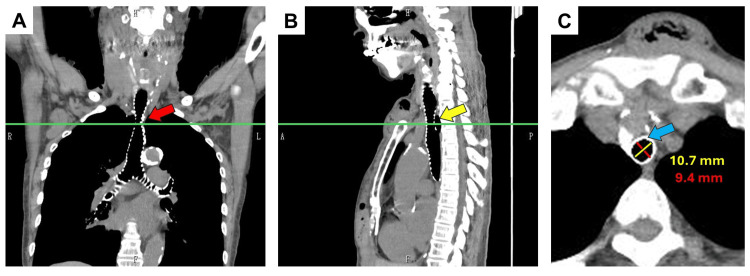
Postoperative computed tomography multi-planar reconstruction image of the present case. A: Coronal image of the trachea. Tracheal stenosis (red arrow) is observed. The green line indicates the level of the narrowest part of the trachea. B: Sagittal image. Tracheal cartilage is present on the dorsal side of the trachea (yellow arrow) at the site of the tracheal stenosis. The green line indicates the level of the narrowest part of the trachea. C: Axial image at the level of the narrowest part of the trachea. The level of the narrowest part is indicated by the green line in images A and B. A complete tracheal ring and a defect in the membranous portion are observed (blue arrow). The narrowest point measured 10.7 mm in the longitudinal diameter (yellow line) and 9.4 mm in the transverse diameter (red line).
